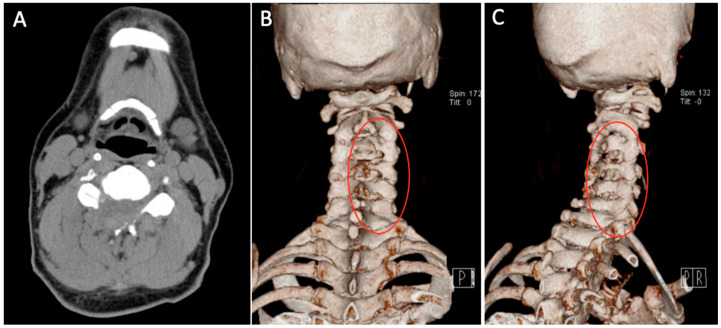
Postoperative axial CT images (A) and CT 3-dimensional reconstruction (B,C). CT = computed tomography. The areas marked by red circles indicate the parts of the cervical spine that were decompressed during surgery.
